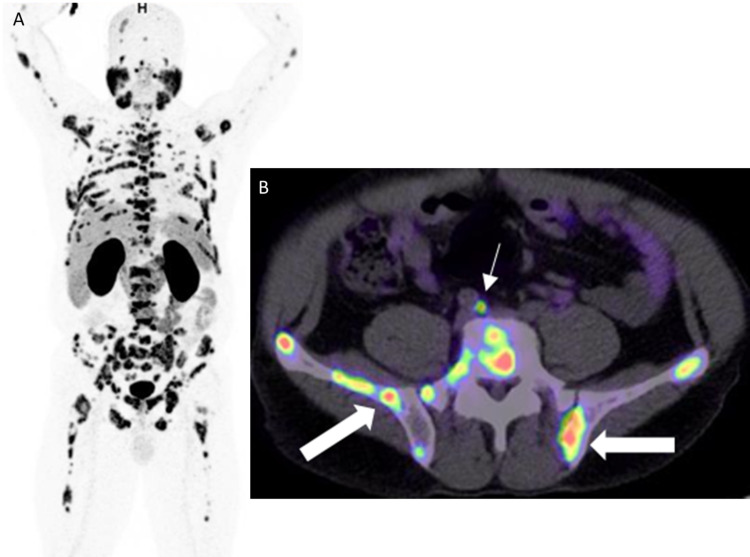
Diffuse bone and iliac lymph node metastases A: 68Ga PSMA PET MIP image shows diffuse bone and iliac lymph node metastases. B: Axial PET/CT image through the pelvis in the same patient shows tracer uptake in the right common iliac lymph node metastasis (white thin arrow) and multiple bone metastases (white thick arrows).
